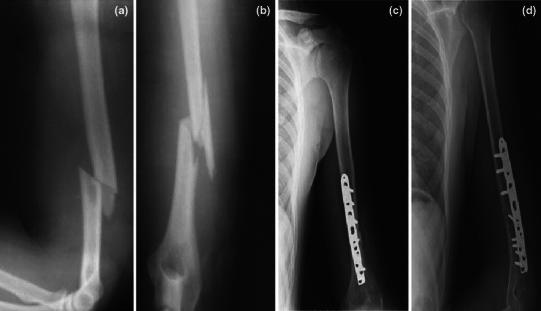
a and b Preoperative X-ray AP and lateral view showing displaced fracture of the shaft of the humerus, left side, in a 26-year-old female patient. c Immediate postoperative X-ray showing plate osteosynthesis with 4.5-mm LCP. d Postoperative X-ray at 12-week follow-up visit showing a well-uniting fracture
